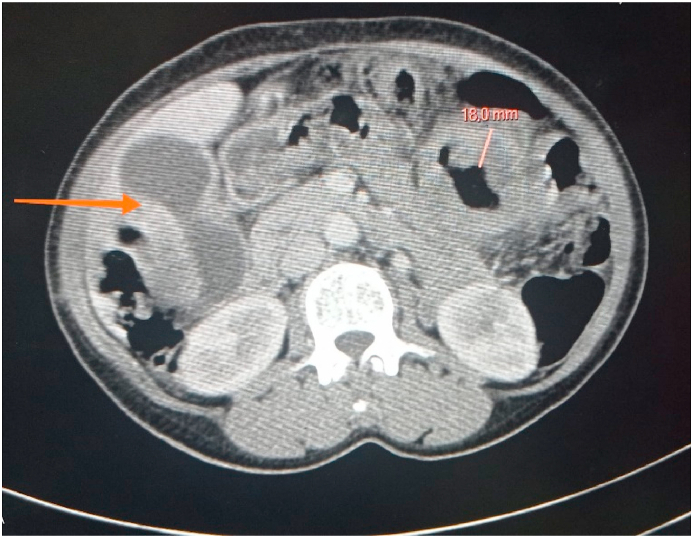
Abdominal CT scan image showing gallbladder and sigmoid colon process.
